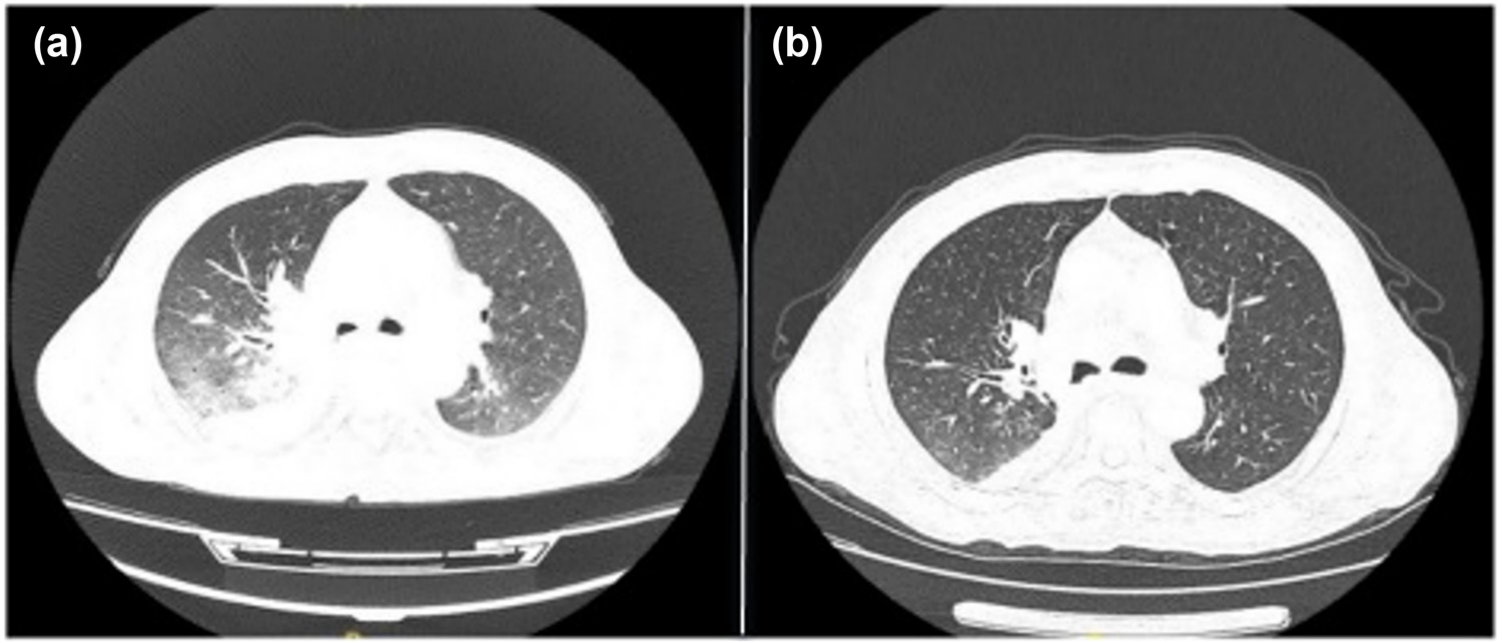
(a) CT scan showing a right hilar mass with features indicative of obstructive pneumonia. (b) Follow-up CT scan exhibits decreased size of the right hilar mass and improved lung consolidation, indicative of treatment response.
